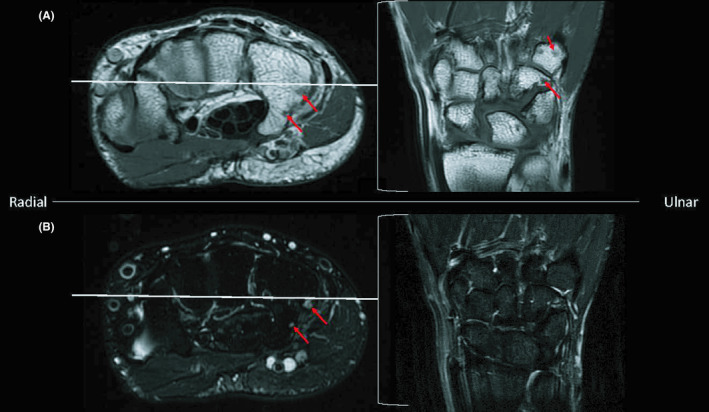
MRI showing T1 hypointense and T2 hyperintense (arrows) 6‐mm lesion in the hook of the hamate, as well as a 3‐mm body in the ulnar body of the hamate and a 4‐mm lesion in the ulnar base of the proximal metacarpal (arrows)
